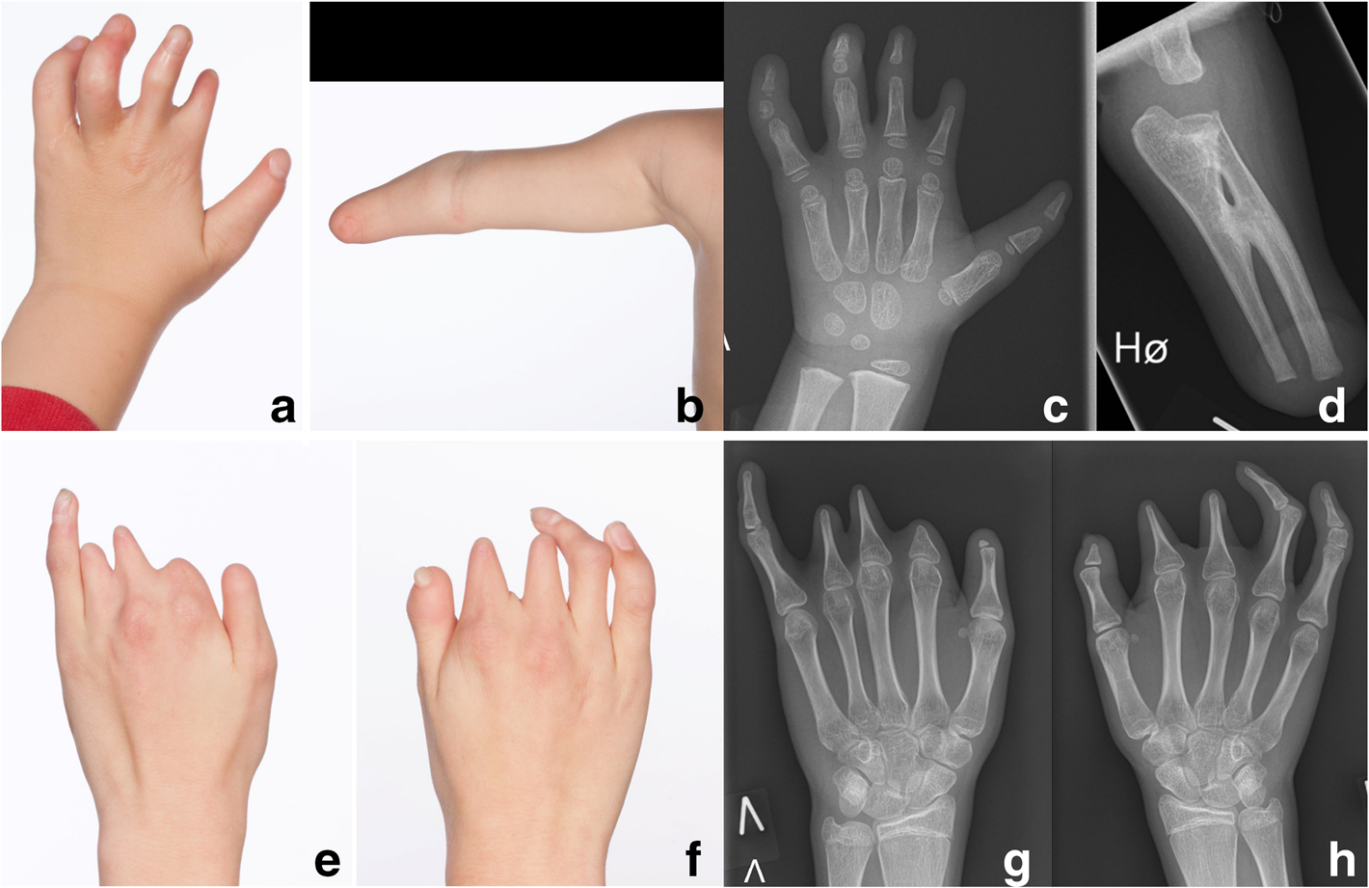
Hands and arm deformities of two patients with Moebius. Clinical photographs and radiographs of the hand and arm of patient no. 1 (a-d) shows terminal transverse congenital deficiency of the right forearm with a radioulnar synostosis, brachydactyly (shortness of fingers) of the five fingers on the left hand, slight syndactyly of the left second and third finger and camptodactyly (flexion contracture of the proximal interphalangeal joints) of the left fourth and fifth finger. Clinical photographs and radiographs of the hands of patient no. 3 (e-h) shows brachydacyly of all ten fingers, slight syndactyly of the left second and third finger, adactyly of the left second finger and camptodactyly of the right fourth and fifth finger
